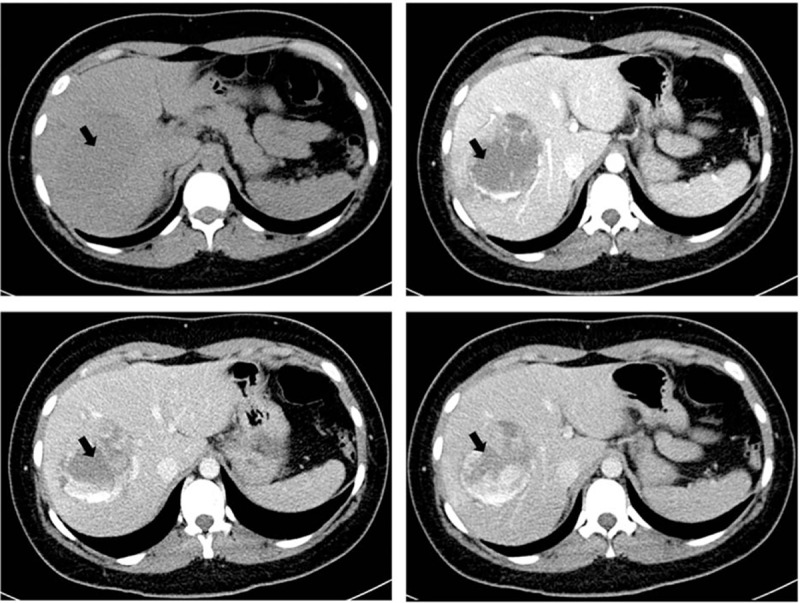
Unenhanced axial computed tomography (CT) scan and enhanced CT scan at arterial, portal, and later phases. Enhanced axial CT showing early leak of contrast material at the arterial phase due to arterial bleeding with increasing leak at the portal and later phases.
